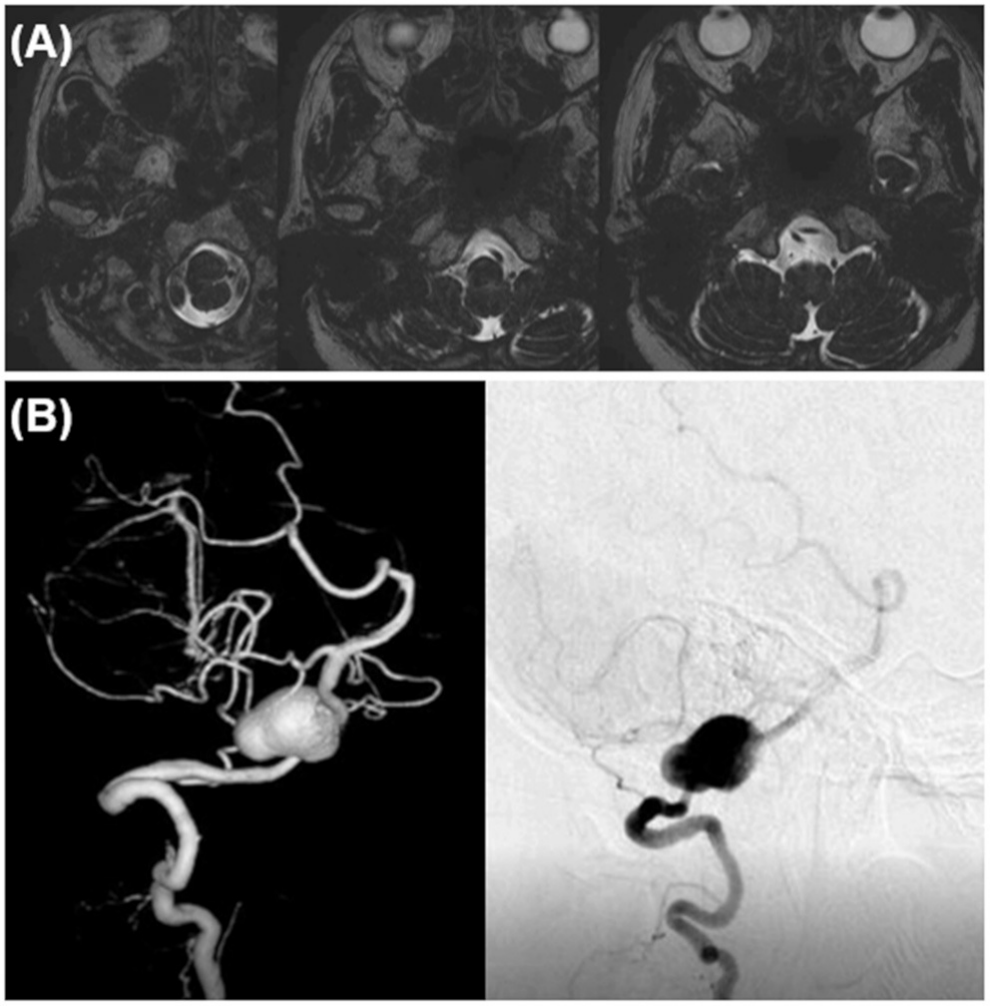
Preoperative image examinations of case 1. (A) Magnetic resonance imaging. (B) Digital subtraction angiography.
